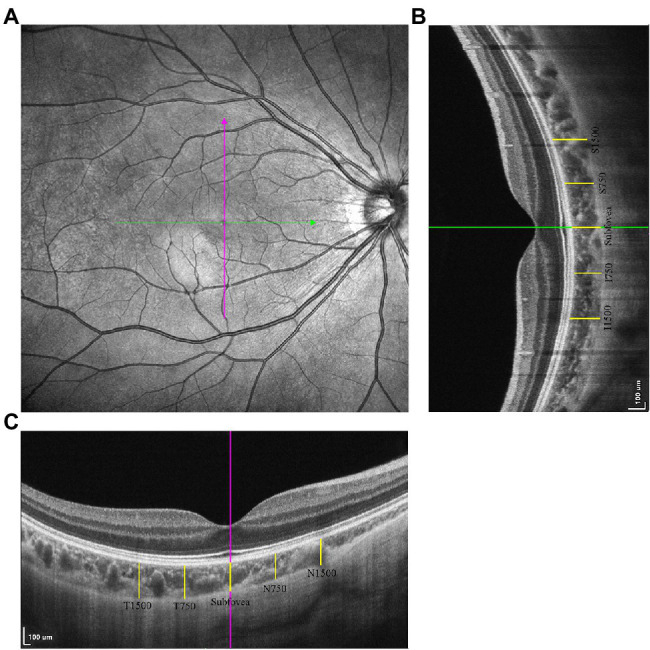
The measurements of choroidal thickness (CT). (A) The horizontal and vertical scan line centering on the fovea. (B) The choroidal thickness measurements in vertical scan. (C) The choroidal thickness measurements in horizontal scan.
